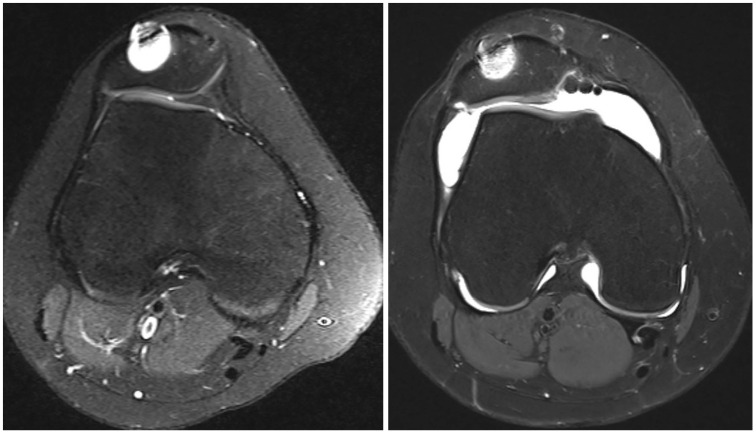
Preoperative axial magnetic resonance imaging (MRI) and postoperative magnetic resonance arthrography (at 21 months) of the patellofemoral joint in a patient who underwent revision medial patellofemoral ligament reconstruction (MRI artifact due to anchor placement in the patella) and trochleoplasty with an unchanged trochlear cartilage status of grade 0 preoperatively and postoperatively.
